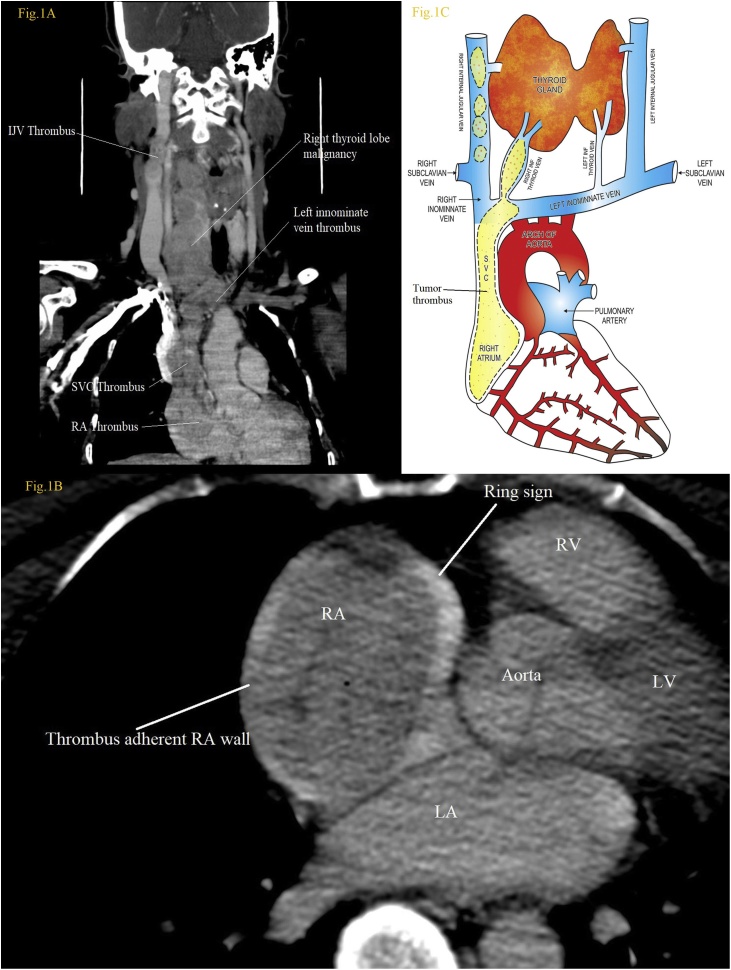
(A) Contrast enhanced CT scan coronal section showing intravenous tumor thrombus extension in the neck vein and thorax. (B) Contrast enhanced CT scan thorax axial image showing tumor thrombus in the RA with Ring sign. (C) Artistic representation of thyroid tumor (brown colored) and intravenous tumor thrombus extension in cervical veins, SVC and RA.
